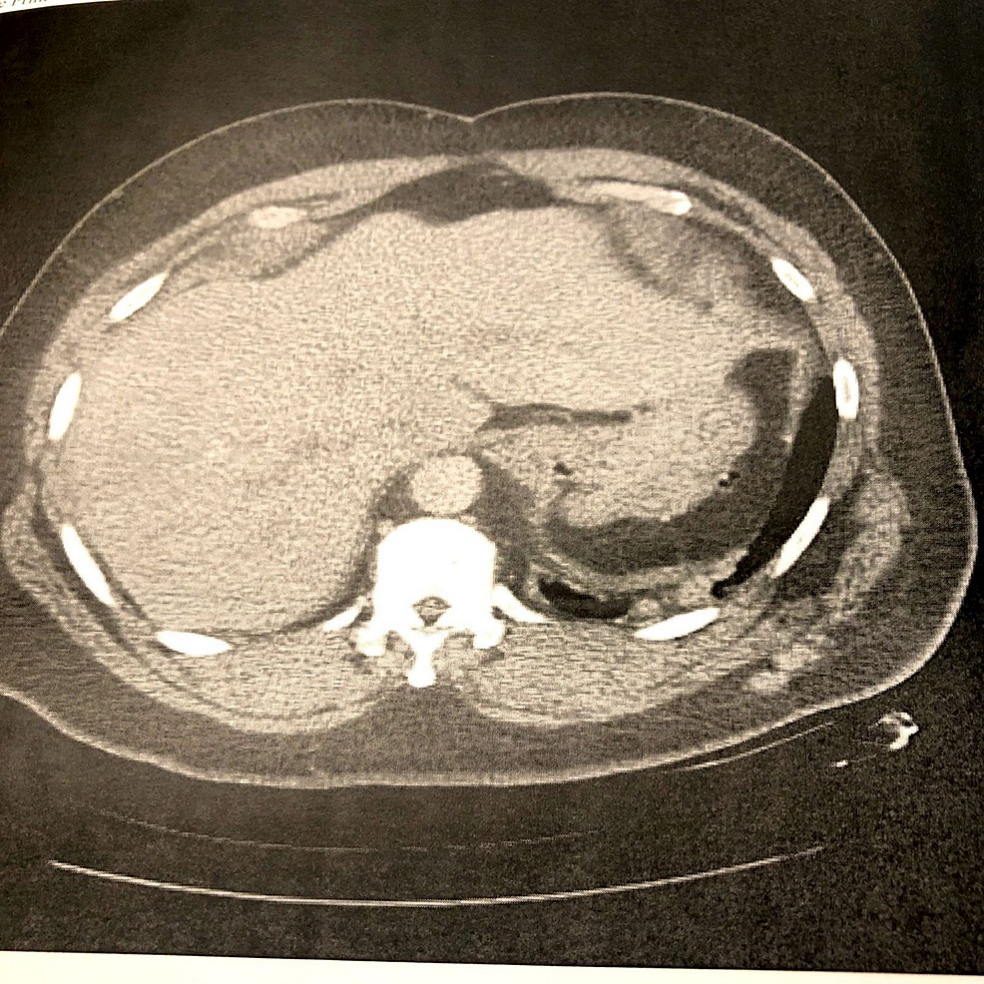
CT abdomen/pelvis w/ contrast.
